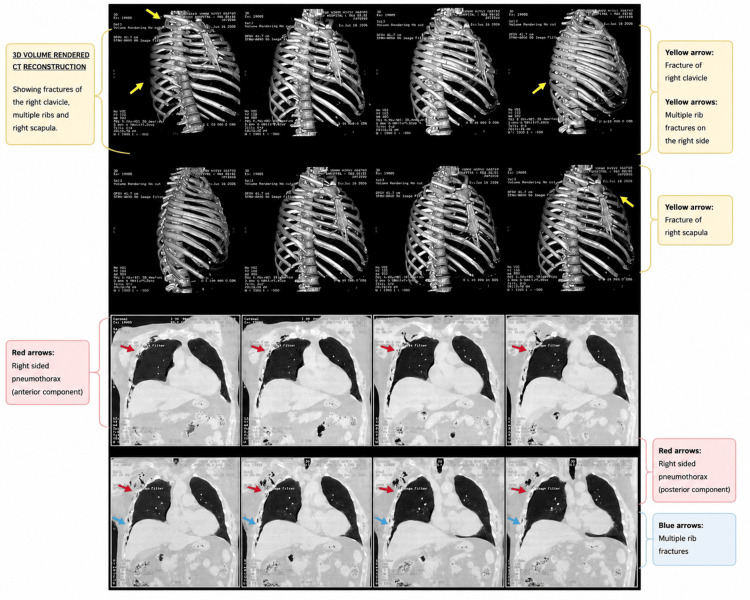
Preoperative CT of the thorax demonstrating the traumatic injuries (a-h) Three-dimensional volume-rendered CT reconstructions demonstrating a displaced fracture of the middle third of the right clavicle (yellow arrow), multiple right-sided rib fractures (yellow arrows), and a comminuted fracture of the right scapular body (yellow arrow). (i-p) Coronal lung-window CT images demonstrating a mild right-sided traumatic pneumothorax (red arrows) with associated subcutaneous emphysema and multiple right-sided rib fractures (blue arrows). CT: computed tomography
